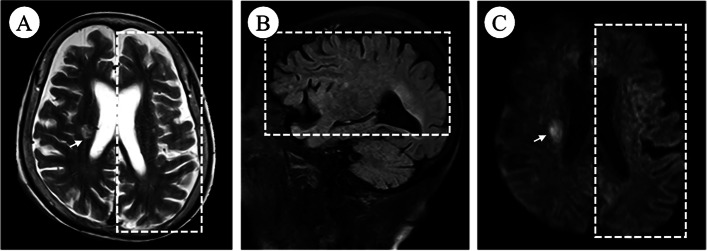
Brain MRI after 4 hours. Brain MRI showed normal signal on axial T2WI (A) and sagittal FLAIR (B), but mild gyral hyperintense on axial DWI (C) in the left brain (dotted square); subacute cerebral infarction near the right lateral ventricle (white arrow with tail)
